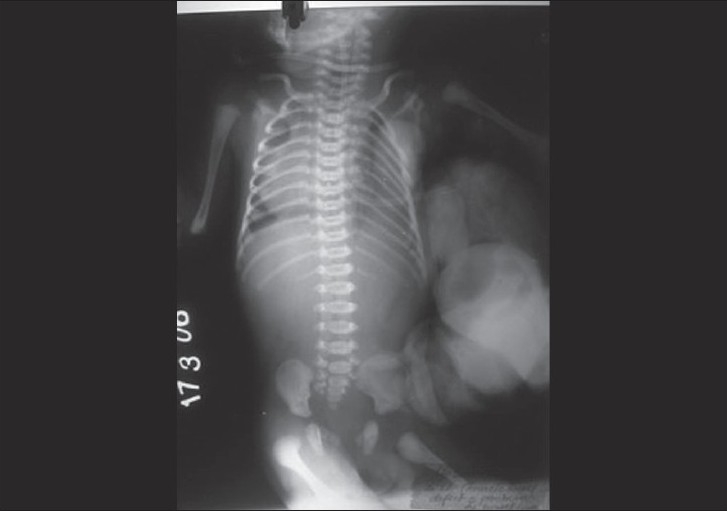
Radiograph showing mediastinal shift and NG tube in stomach lying outside the chest wall
